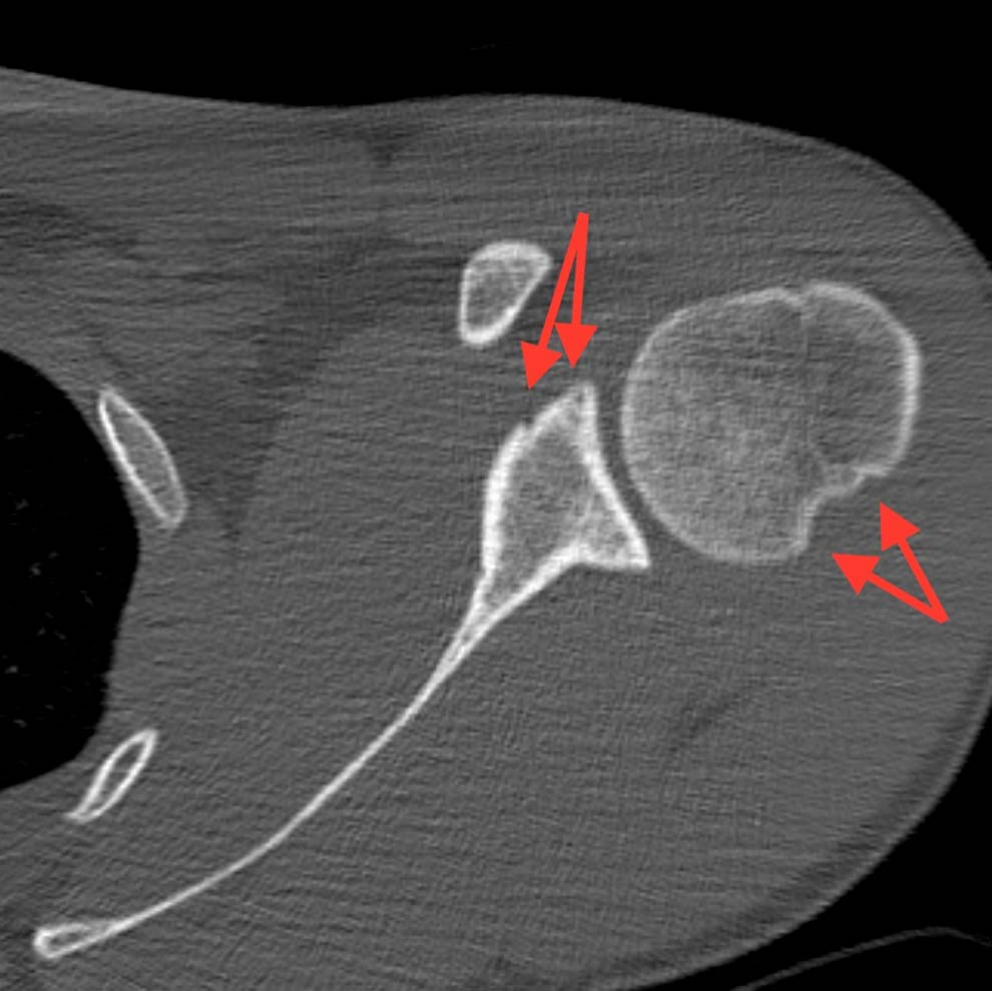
Computed tomography scan of the left shoulder, axial cross-sectional image, demonstrates mild compression, or Bankart lesion, of the anterior glenoid fossa over a 1.1-cm area (upper arrows, anterior) and a Hill-Sachs lesion measuring 1.4 cm × 0.3 cm on the humeral head (lower arrows, posterior).
